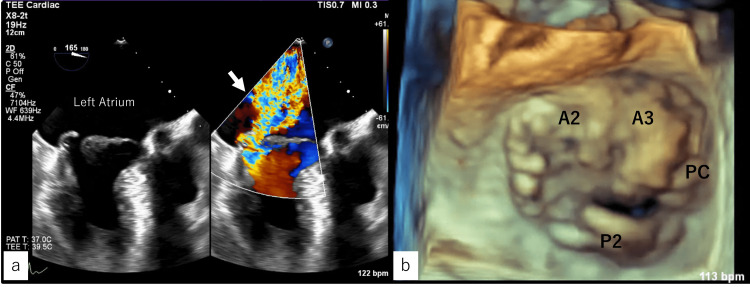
Preoperative transthoracic echocardiography (a) Transthoracic echocardiography demonstrating severe mitral regurgitation (arrow) with dilated left atrium. (b) Three-dimensional echocardiography showing prolapse of P2, A3, and the posterior commissure (PC).
